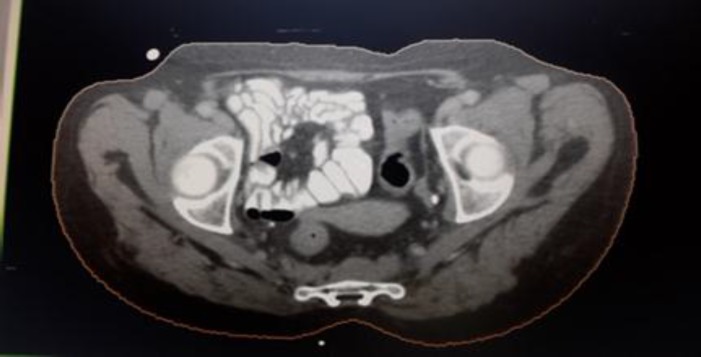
CT scan showing presacral mass
